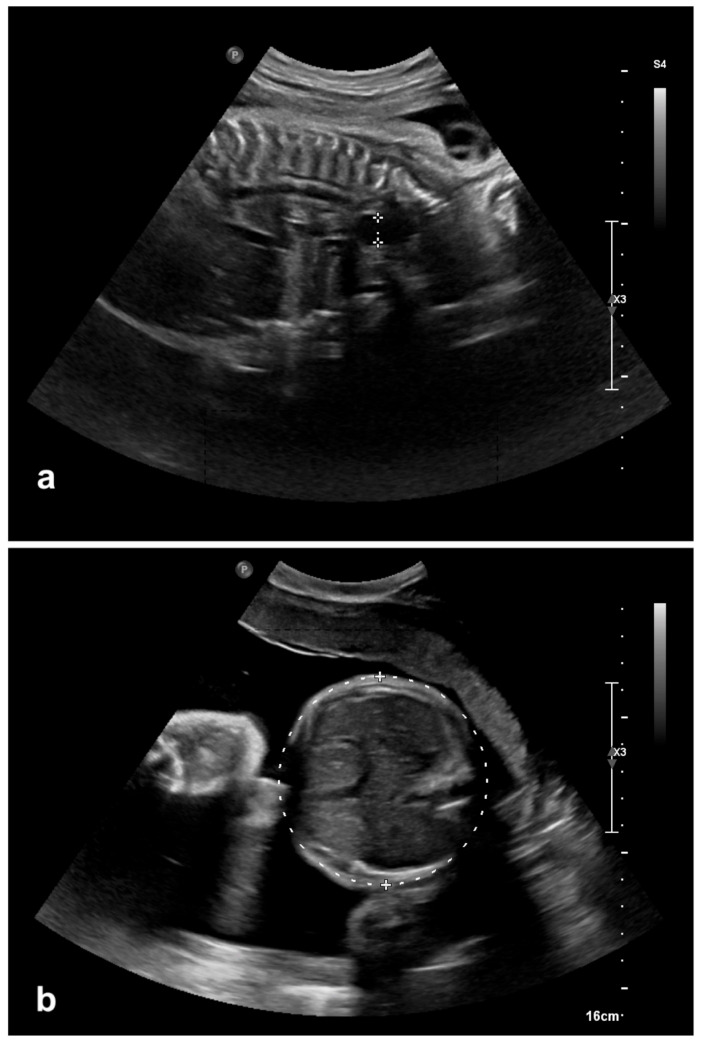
(a) “Pouch sign”—Prevertebral fluid accumulation inside the region of the neck/mediastinum, possibly suggesting esophageal atresia in gestational week 31. (b) Fetal ultrasound showing the absence of the stomach intraabdominally in gestational week 31.
